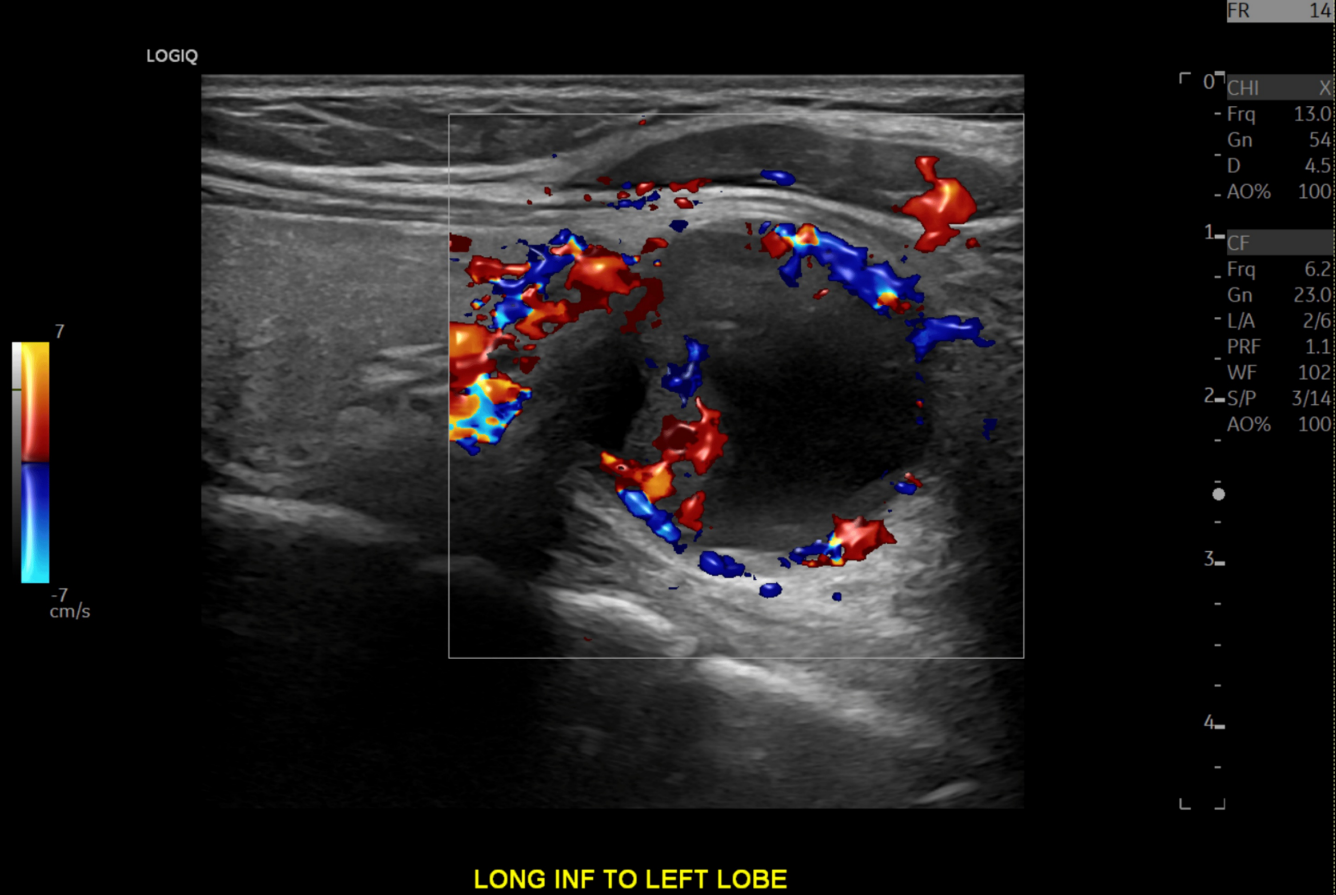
Thyroid ultrasound The thyroid ultrasound with color Doppler imaging showing a partially exophytic mixed solid and cystic nodule along the left inferior thyroid lobe with peripheral and septal blood flow.
